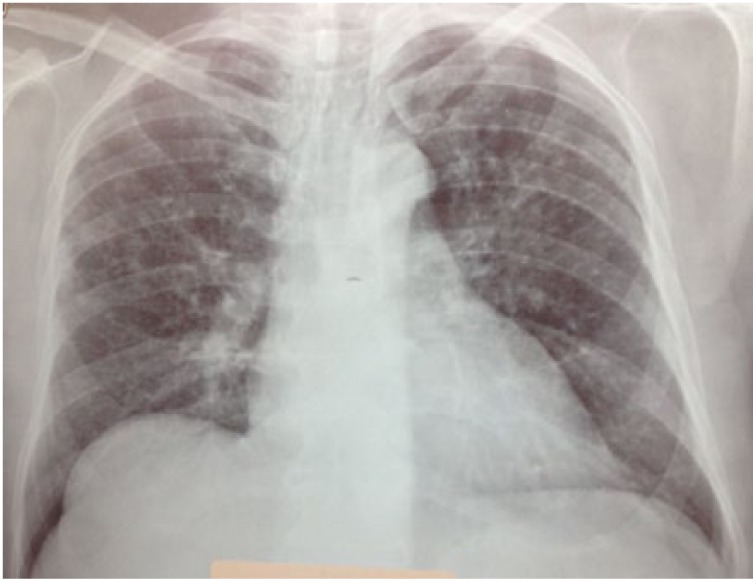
The chest x-ray during anti-TNF treatment (August 2012).
